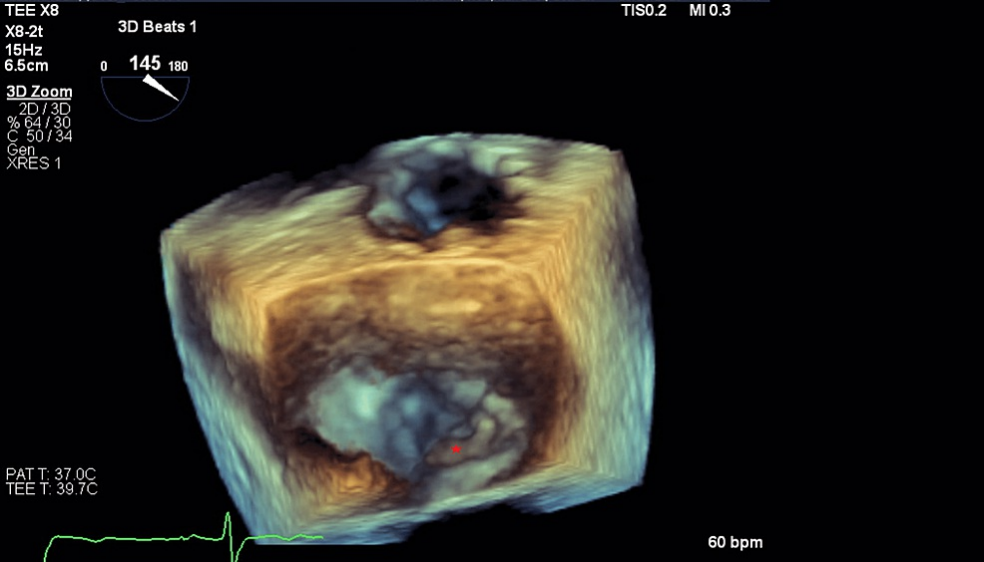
Three-dimensional (3D) image of TEE showing echo density on the posterior mitral valve leaflet (star). TEE: transesophageal echocardiogram
